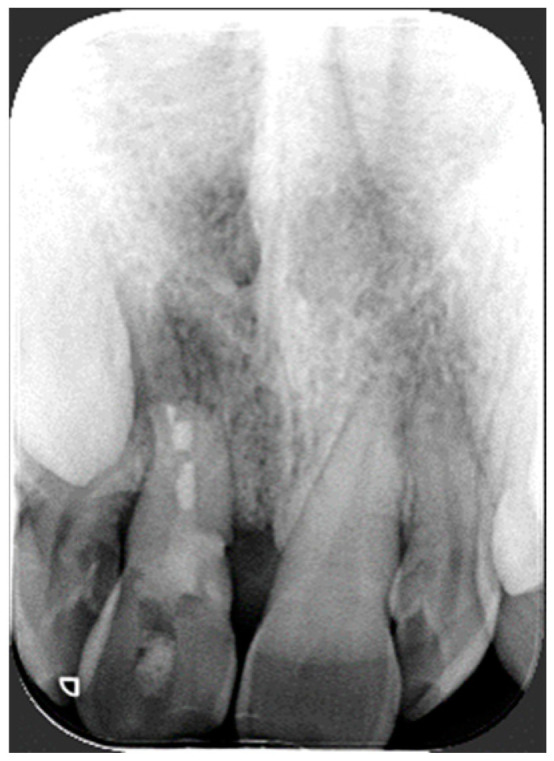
Periapical radiography of the 1.1 tooth after intentional reimplantation and the removal of the splint.
